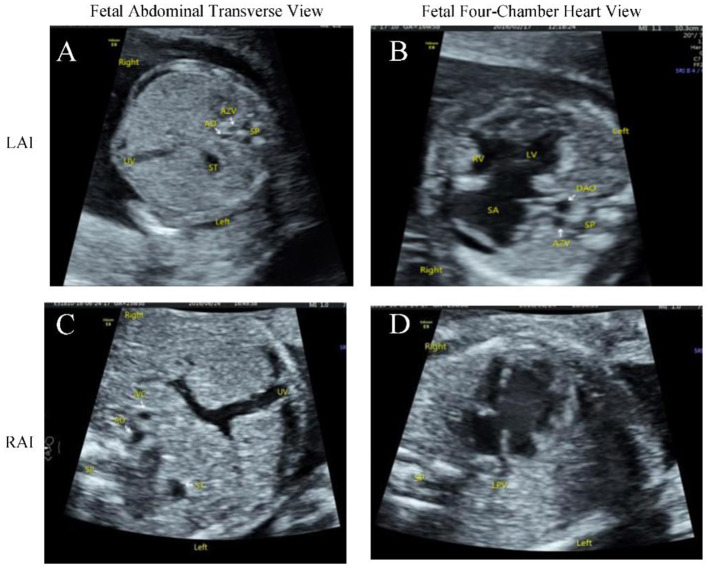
Prenatal ultrasound images of fetal left atrial isomerism (LAI) (A, B). (A) Transverse abdominal view showed the stomach bubble located in the left abdomen with absence of the inferior vena cava. The abdominal aorta was positioned anterior to the spine, with a dilated azygos vein visible posterior and to the right of the aorta. (B) Four-chamber view demonstrated significant cardiomegaly, a common atrium, and right-looping ventricle. The central crux of the heart was absent with only a common atrioventricular valve observed opening and closing. The descending aorta was located anterior to the spine, with a dilated azygos vein visible posterior and to the right of the aorta. Prenatal ultrasound images of fetal right atrial isomerism (RAI) (C, D): (C) Transverse abdominal view showed the stomach bubble in the left abdomen, with the abdominal aorta and inferior vena cava juxtaposed to the right of the spine. The inferior vena cava was located anterior and to the right of the abdominal aorta. (D) Four-chamber view showed the heart positioned in the right hemithorax, discordant with the stomach bubble location. A complete atrioventricular septal defect was present, with the ventricles exhibiting a univentricular morphology dominated by right ventricular characteristics.
